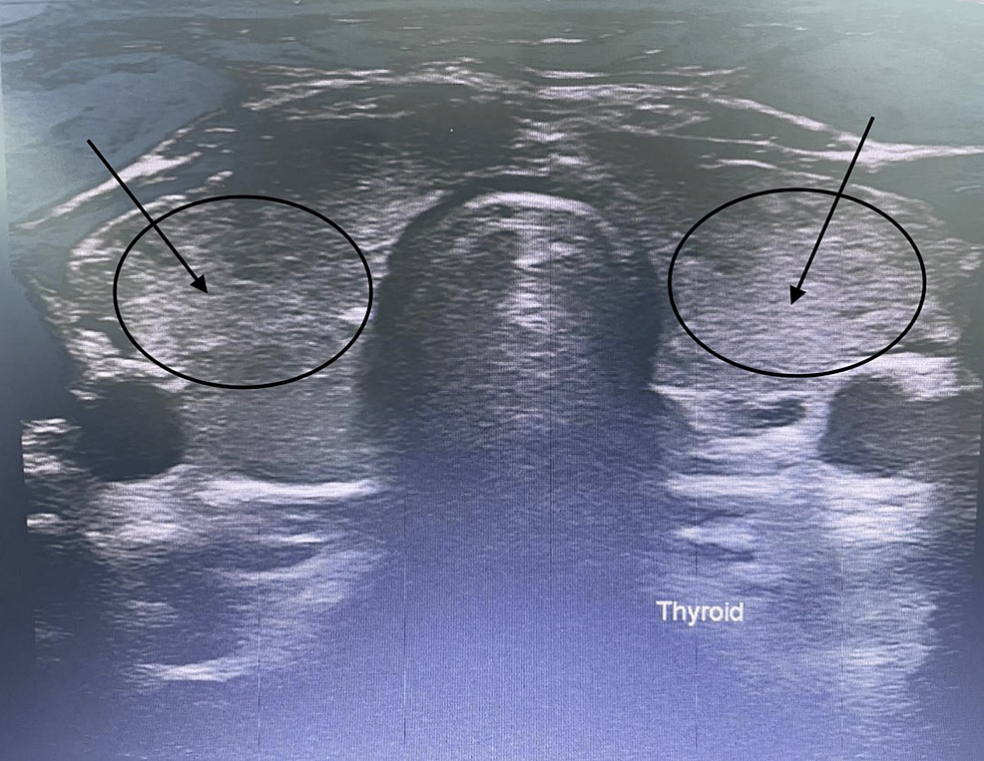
Ultrasound image of the thyroid gland. Heterogenous echogenicity of the thyroid can support hypothyroid findings.
